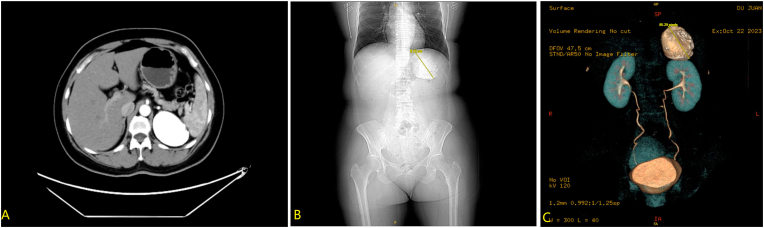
Preoperative CT reveals a calcified lesion in the adrenal region, measuring approximately 8.5 × 7.0 × 5.0 cm, with no enhancement on contrast scan.
